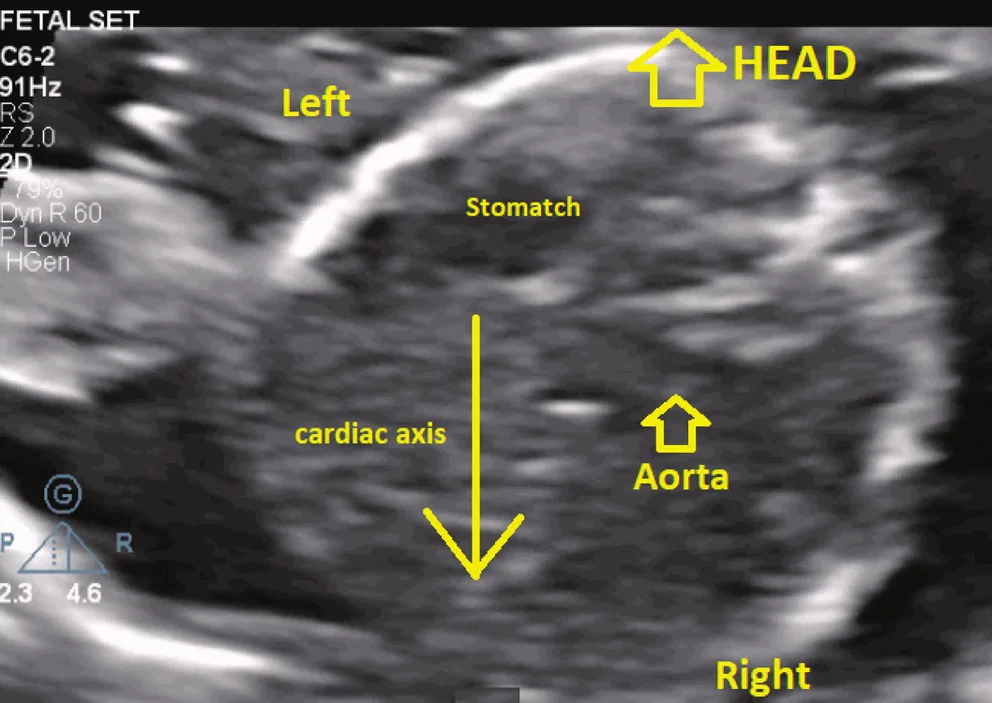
Axial fetal echocardiographic image demonstrates situs solitus with dextrocardia, showing rightward cardiac apex orientation.
